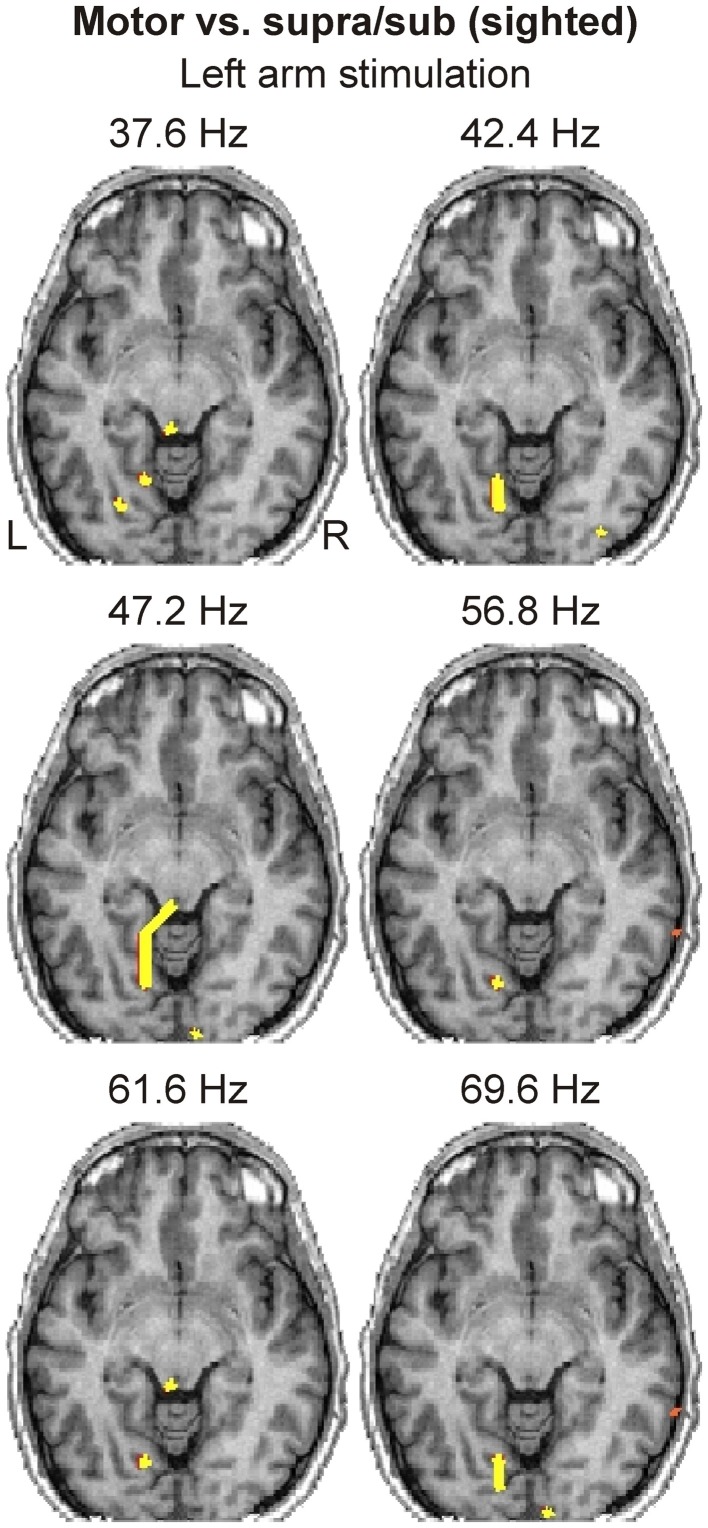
Spatio-frequency activation maps of a sighted subject derived from “Motor vs. Supra/Sub” contrast are shown for left arm stimulation at six frequencies (indicated on top of each image). The axial slice of MRI best covering the visual cortex activations is shown. Yellow contours encompass regions with statistically significant (*P* < 0.0001) increases in activity.
